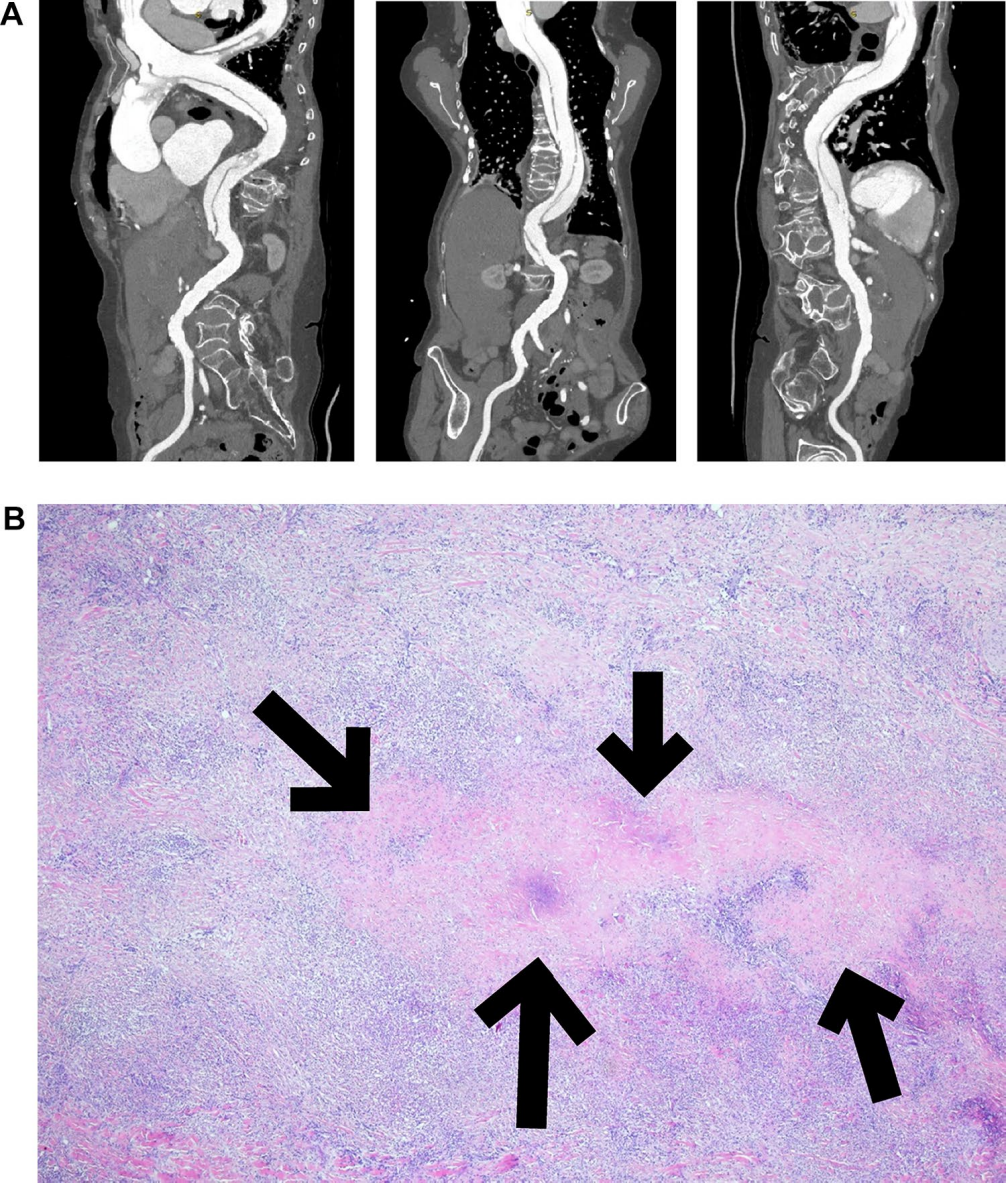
A 46-year-old woman with GPA diagnosis who presents a type A aortic dissection 24 years later. A: CT-scan showing aortic dissection, B: granulomatous vasculitis with chronic inflammation, multiple focus of collagenous necrosis (arrows) without giant cells (courtesy of Jean-Claude Pache, MD)
